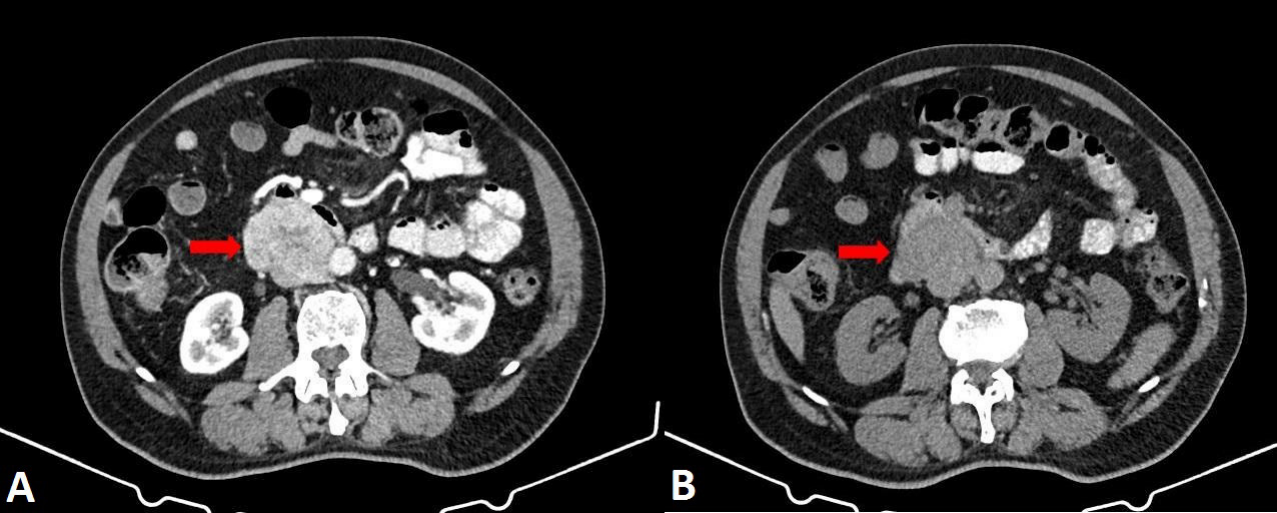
Abdominal computed tomography scan, axial views. Red arrows indicate the large (70 x 60 x 50 mm; longitudinal x transverse x anteroposterior) solid, heterogeneous, expansile mass with lobulated contours (images A and B), located in the median and right paramedian retroperitoneal region, adjacent to the posterior and inferior wall of the third portion of the duodenum, with no clear cleavage plane from the inferior vena cava.
